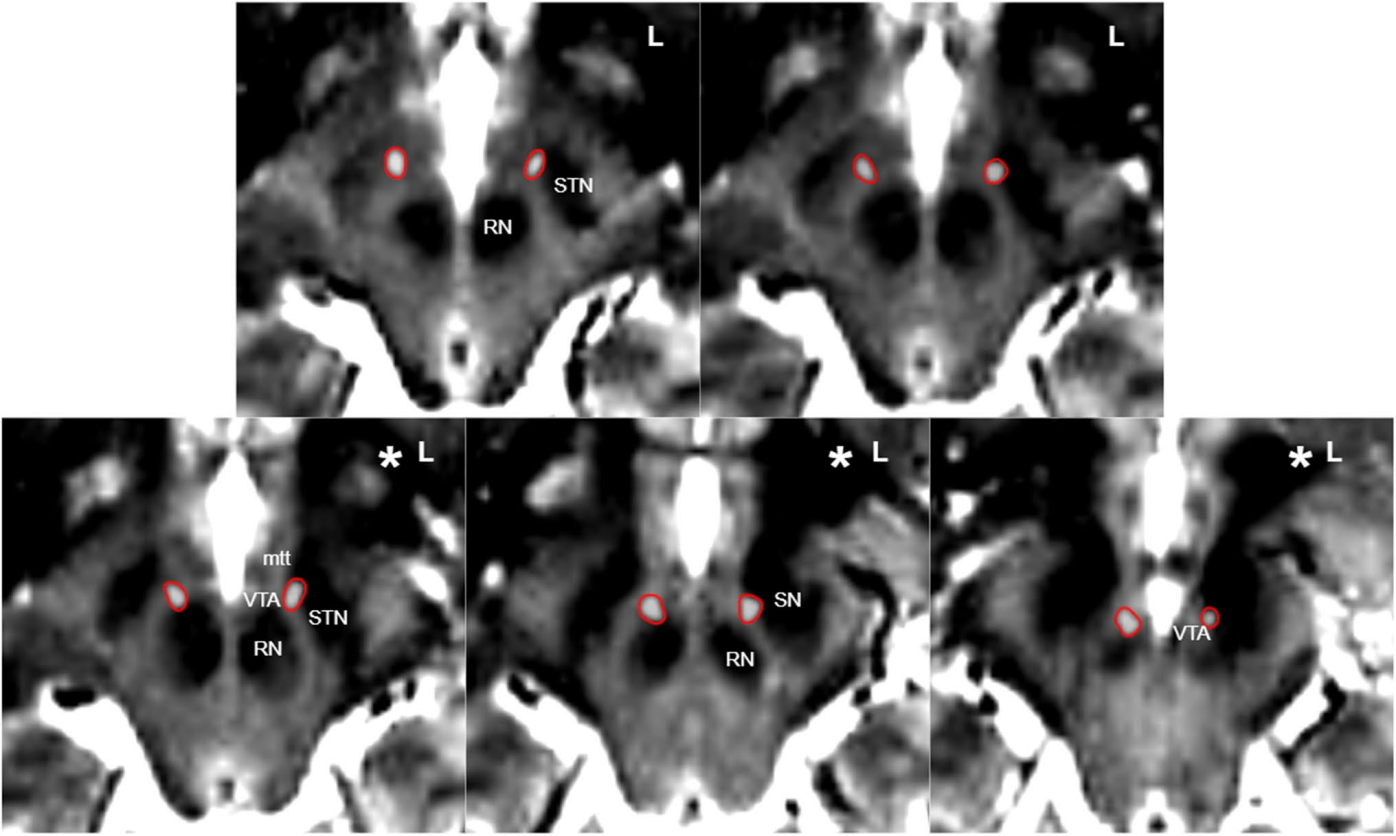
Achieved electrode positions in the lateral ventral tegmental area (VTA). Fusion of postoperative CT (electrode artifacts white dots with red lining) with preoperative T2W MRI. Electrodes are located in the corridor between the mammillothalamic tract (mtt), the red nucleus (RN), and subthalamic nucleus(STN)/substantia nigra (SN) and end with their tips in the VTA in front of the RN. Potentially the left electrode is slightly closer to the STN. * indicates estimated levels of stimulation
